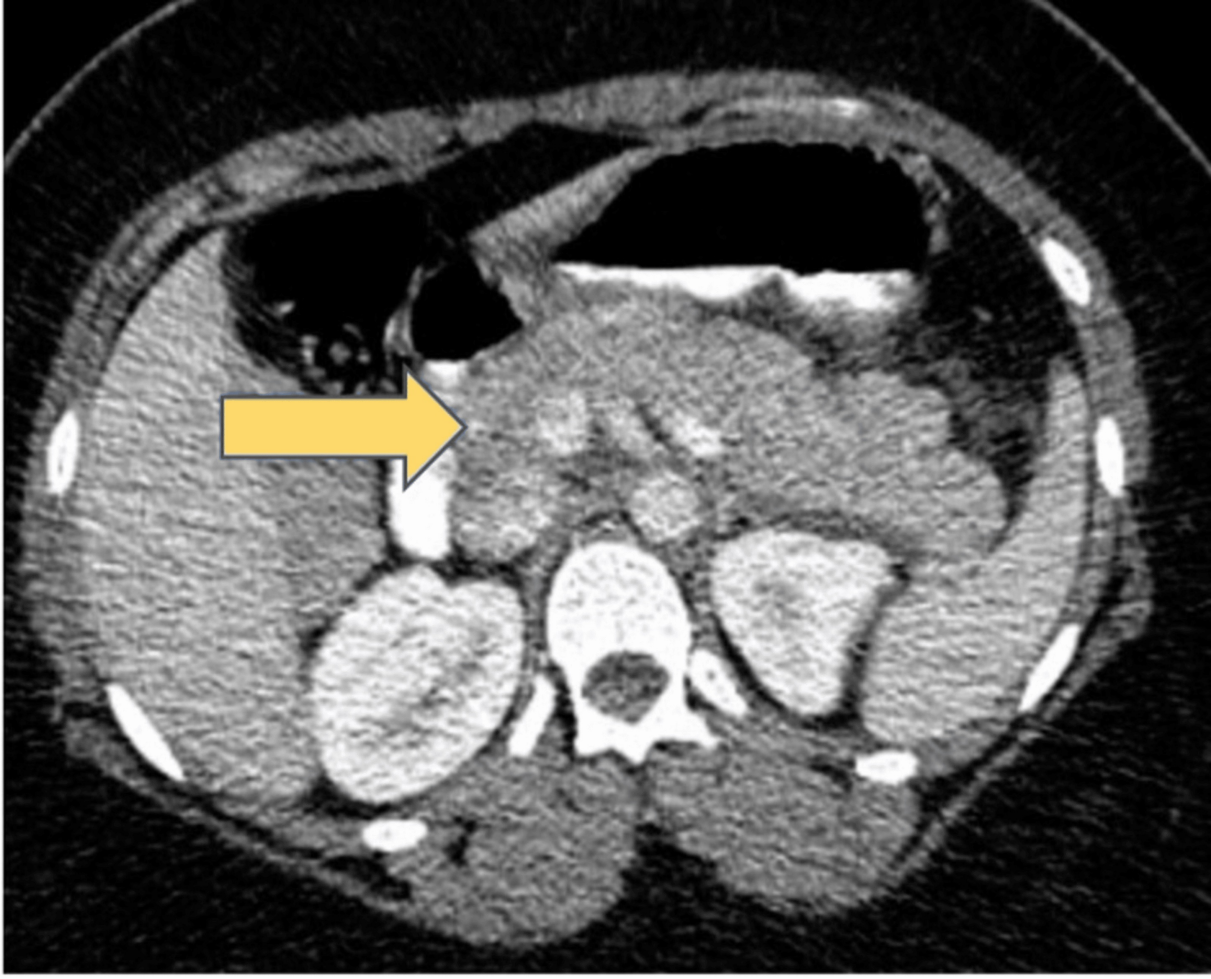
CT abdomen showing fullness of the pancreas with moderate peripancreatic fat stranding and edema [as shown by arrow].
